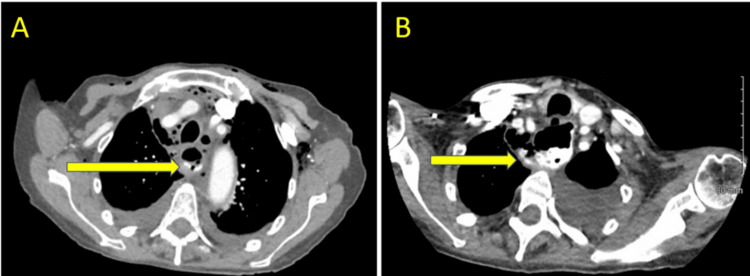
Case 1: CT scans showcasing esophageal leak with pleural effusion on (A) POD 3 and (B) POD 5. POD: Postoperative day.
